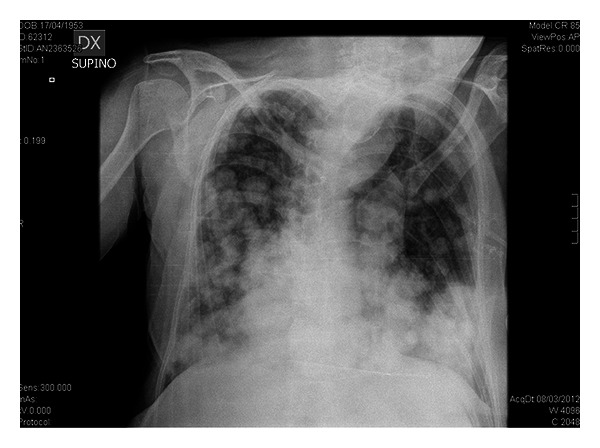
Nodular lesion at chest X-ray. Chest radiography showing bilateral nodular lesions, above all in lower lung fields.
